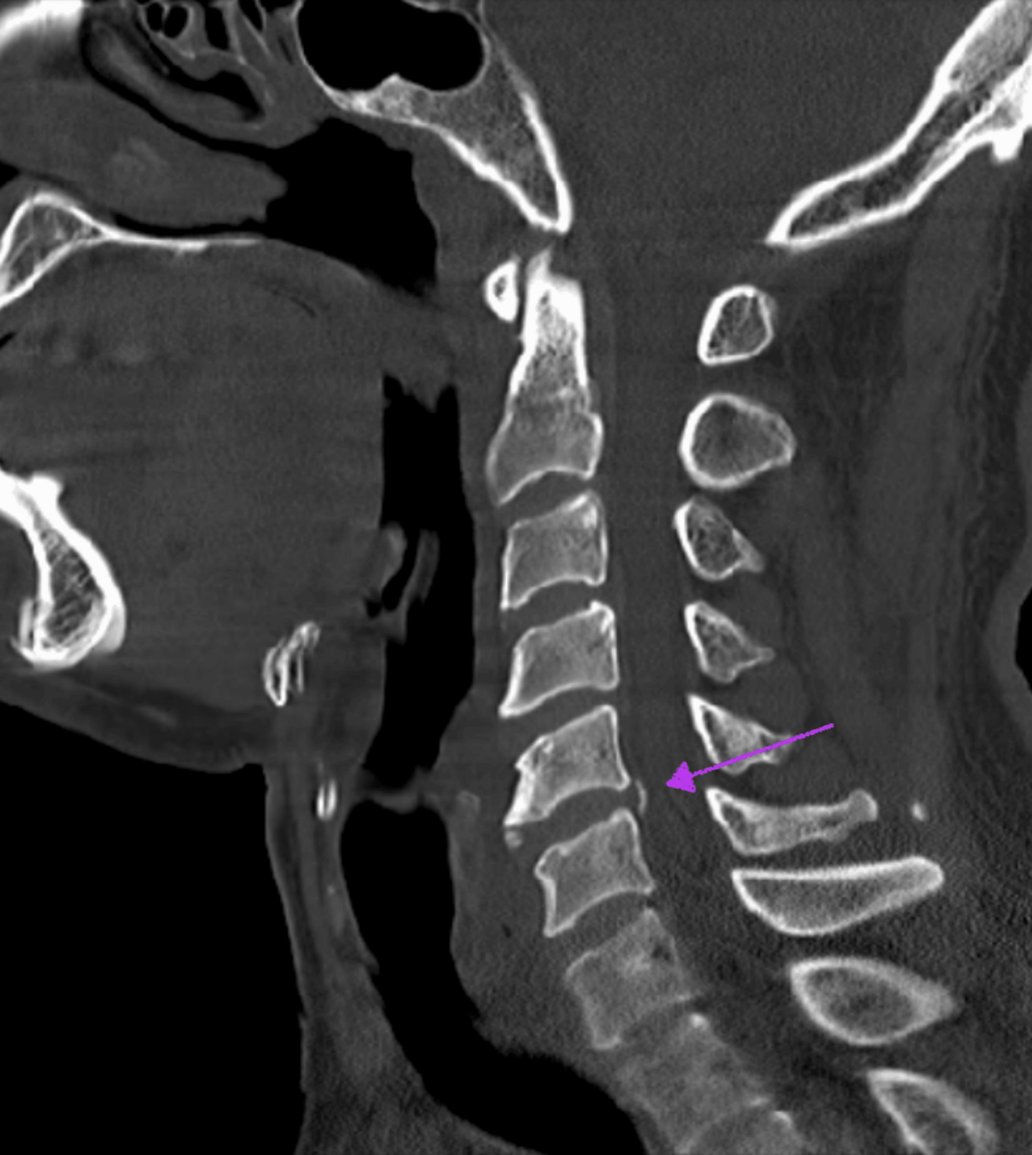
CT showing incidental calcification of the posterior longitudinal ligament (purple arrow) There is no evidence of dural ectasia, which is a more classic spinal manifestation of MFS. MFS, Marfan syndrome
